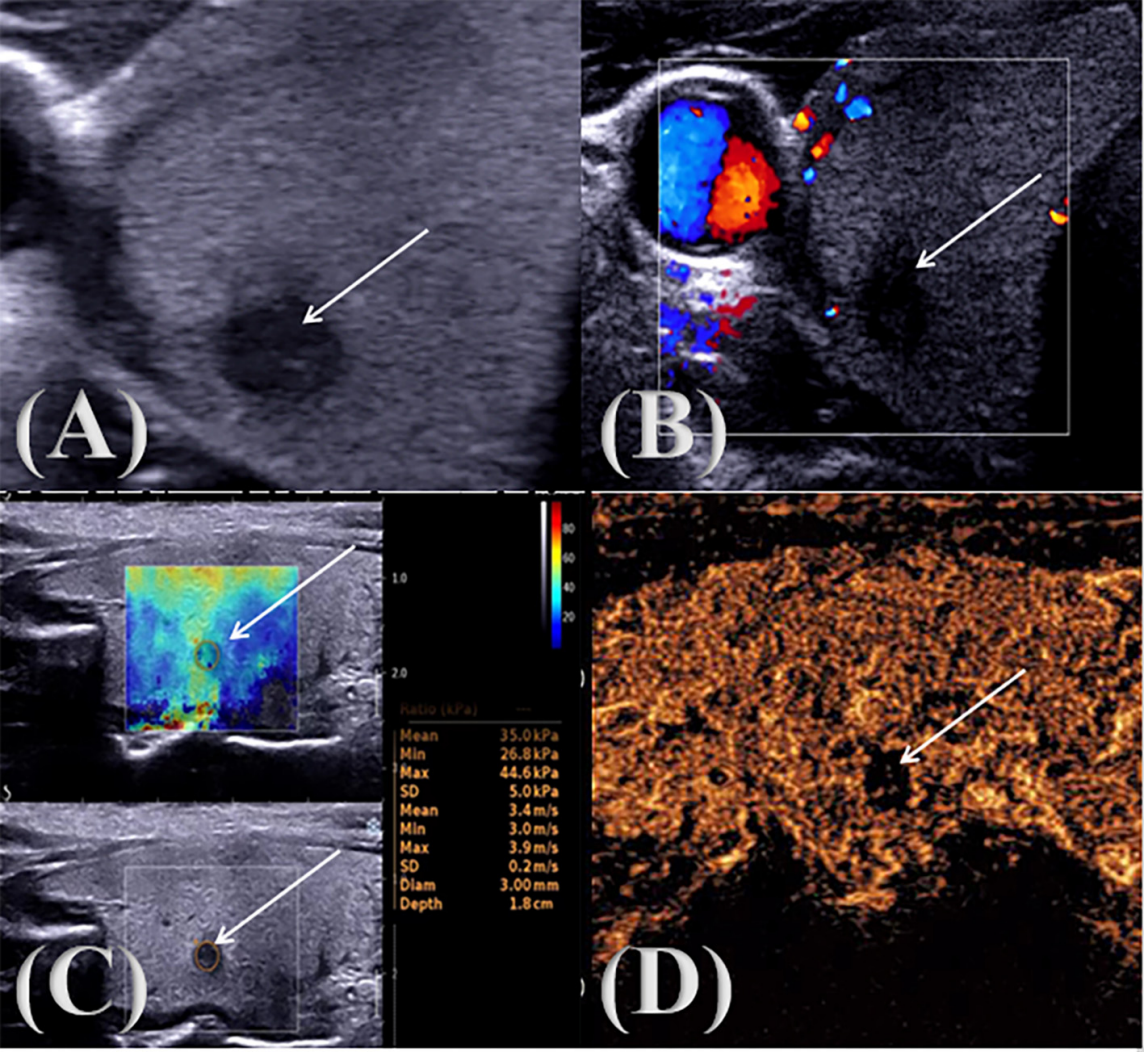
PTC multimodal ultrasound image. ↑: Lesion (A) two-dimensional gray scale ultrasonic image; (B) CDFI audio image; (C) SWE image; (D) CEUS audio image.
